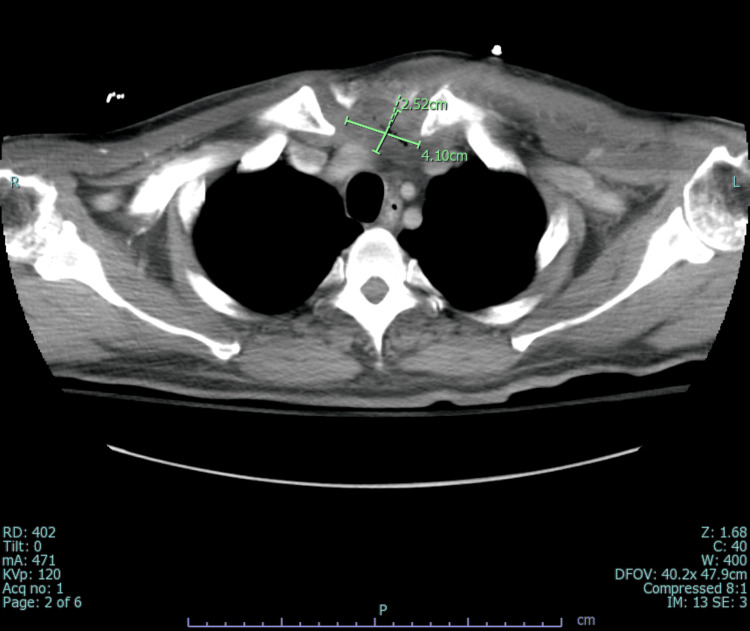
Computed tomography scan (axial view) showing a substernal anterior mediastinal abscess.
